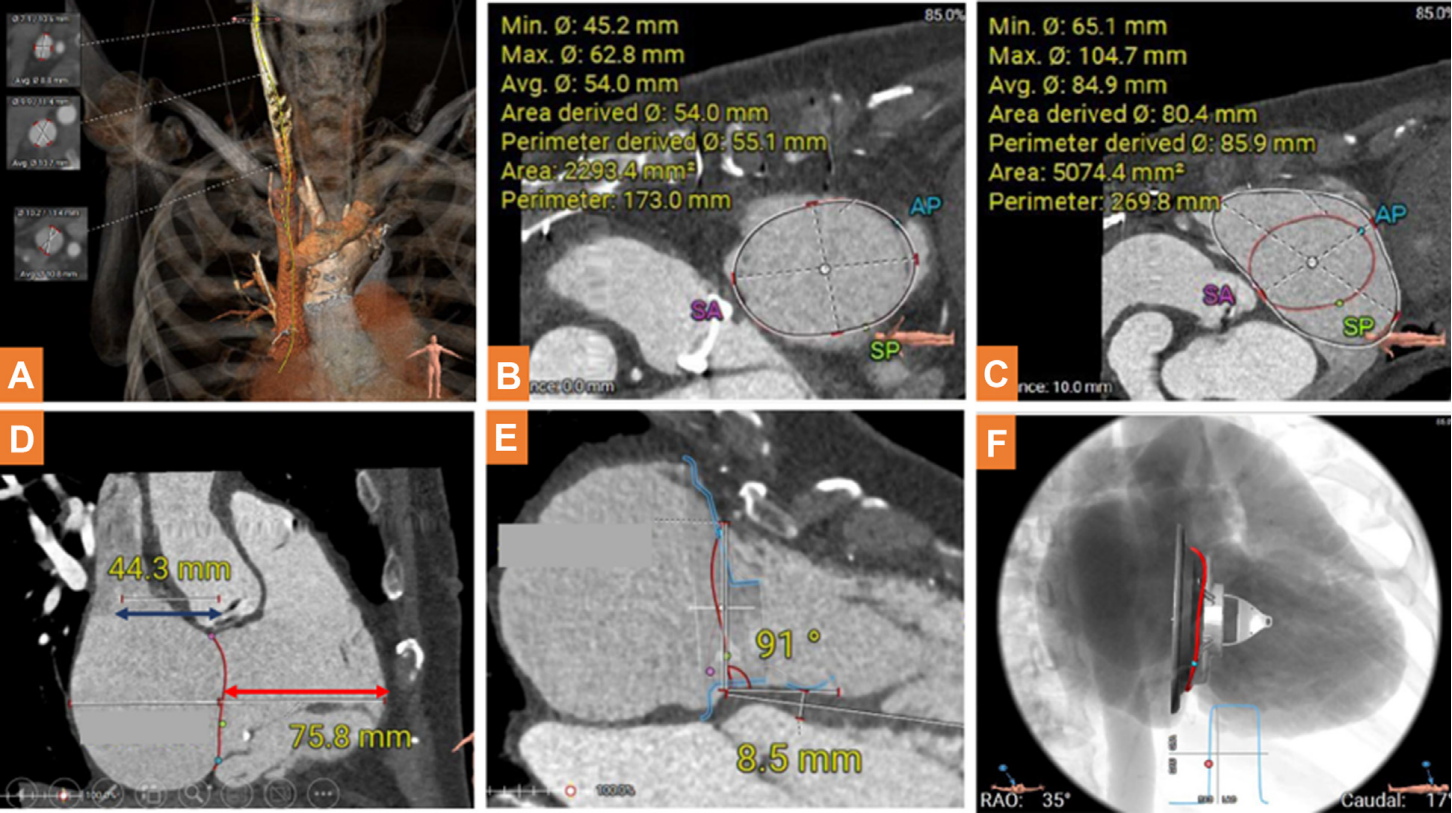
Preprocedural Computed Tomography Assessment
